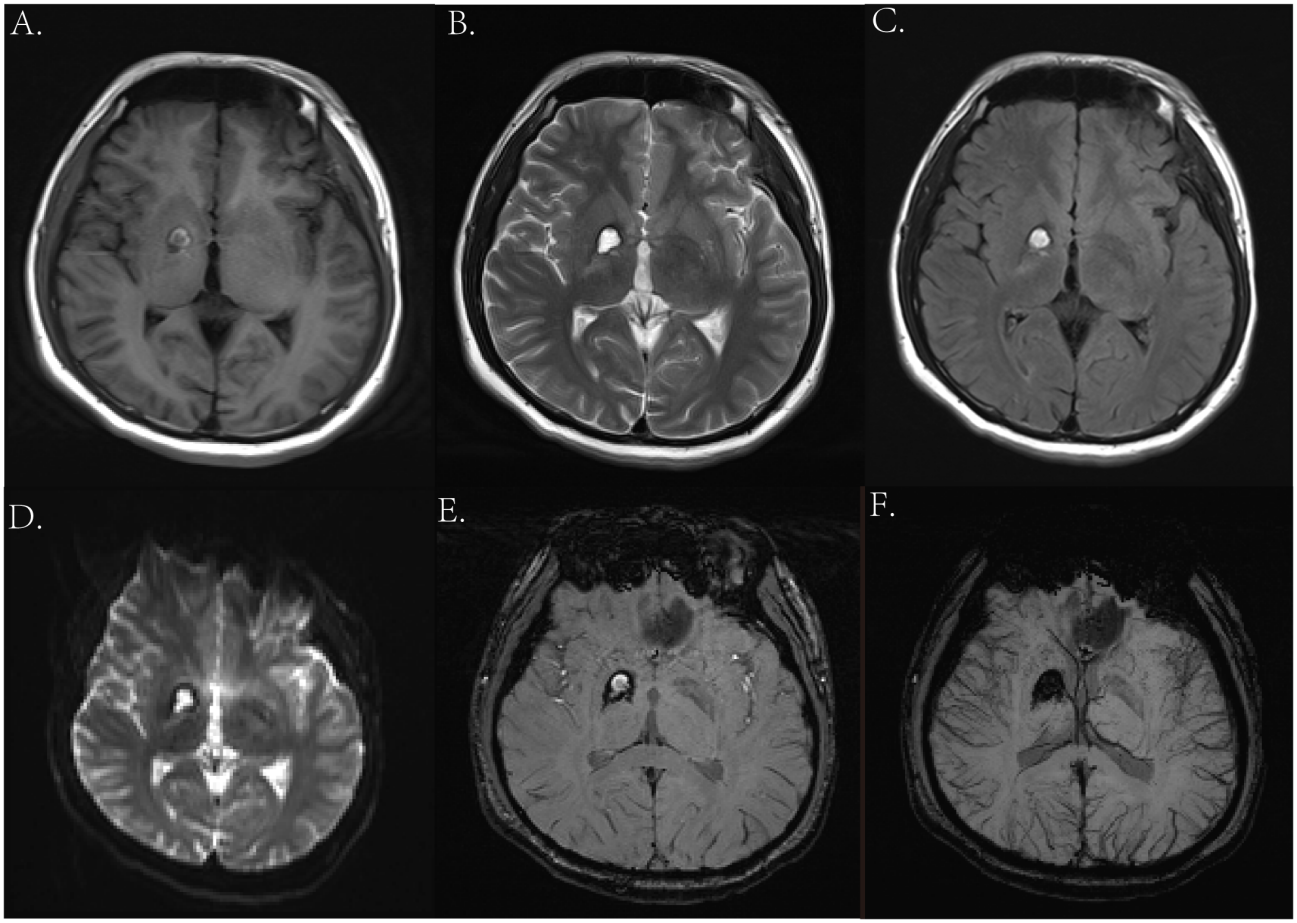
Magnetic resonance imaging (MRI) from previous admission 2 years ago. (A) Axial T1-weighted image. (B) Axial T2-weighted image. (C) Axial T2-FLAIR image. (D) Axial DWI image. (E, F) Axial SWI image.
